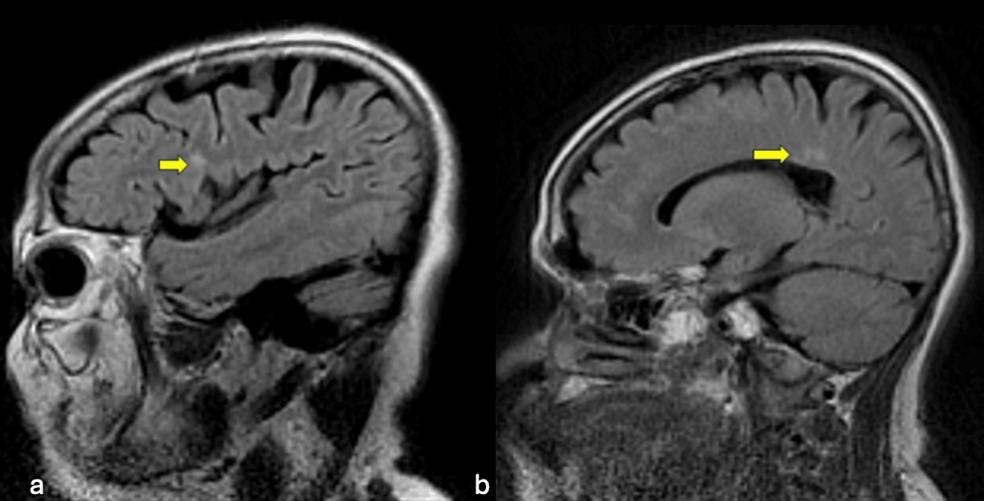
Sagittal T2 fluid attenuated inversion recovery (FLAIR) images (a & b) showed hyperintense periventricular white matter lesion (yellow arrow).
